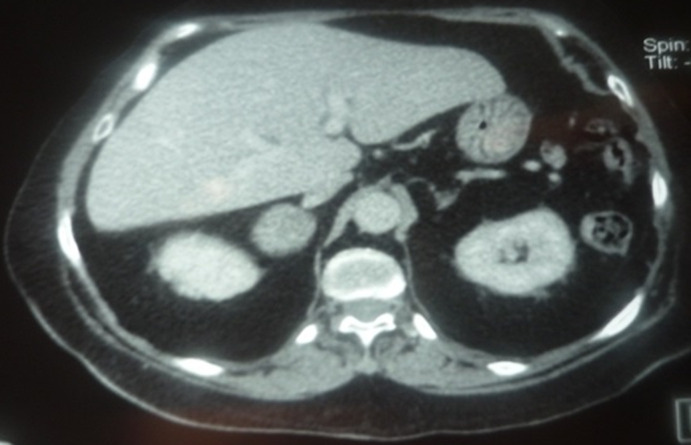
adrenal CT scan, tumor mass at the right adrenal, measuring 46 x 36mm, the left adrenal was not hyperplastic
